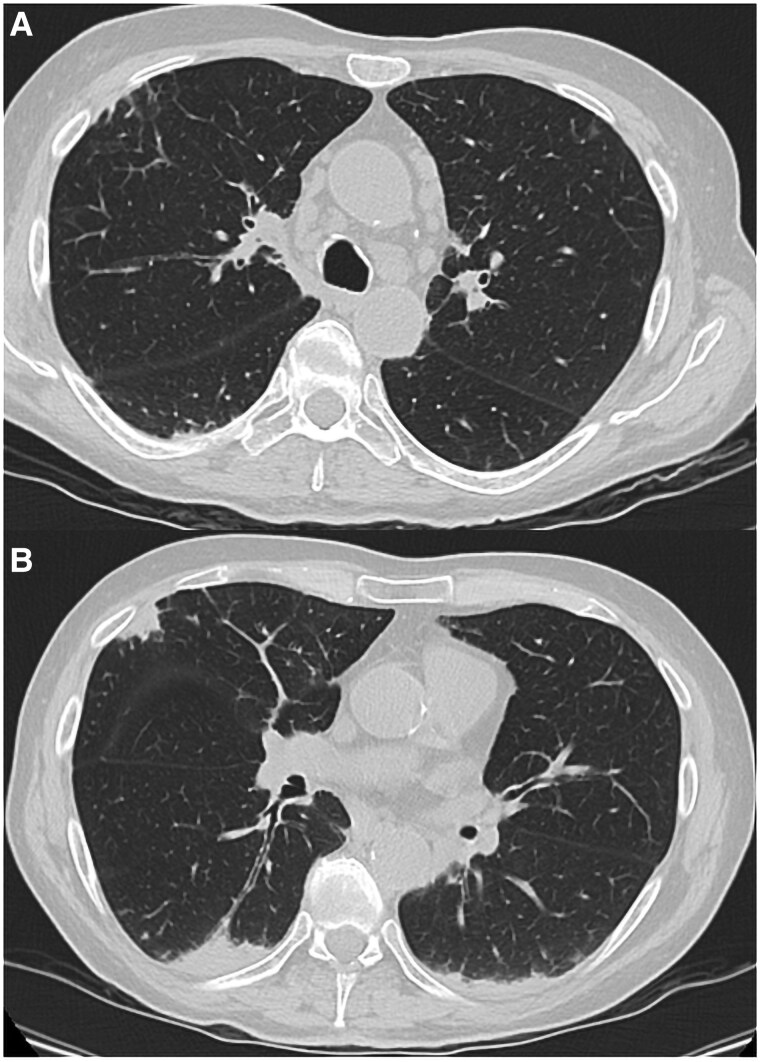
Computed tomography scan showing numerous enlarged mediastinal lymph nodes (*A*) and subpleural thickening of the posterior and medium right lobes and the posterior left lobe (*B*).
